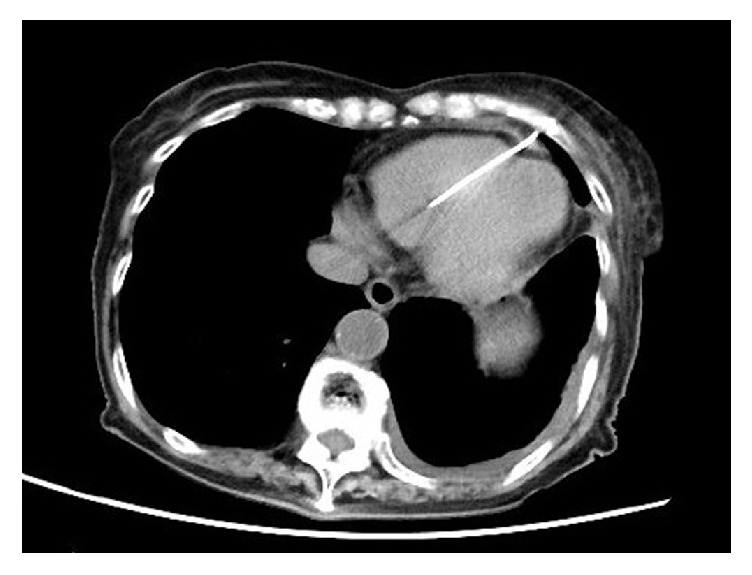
Chest CT reveals perforation of the right ventricle by the lead.
